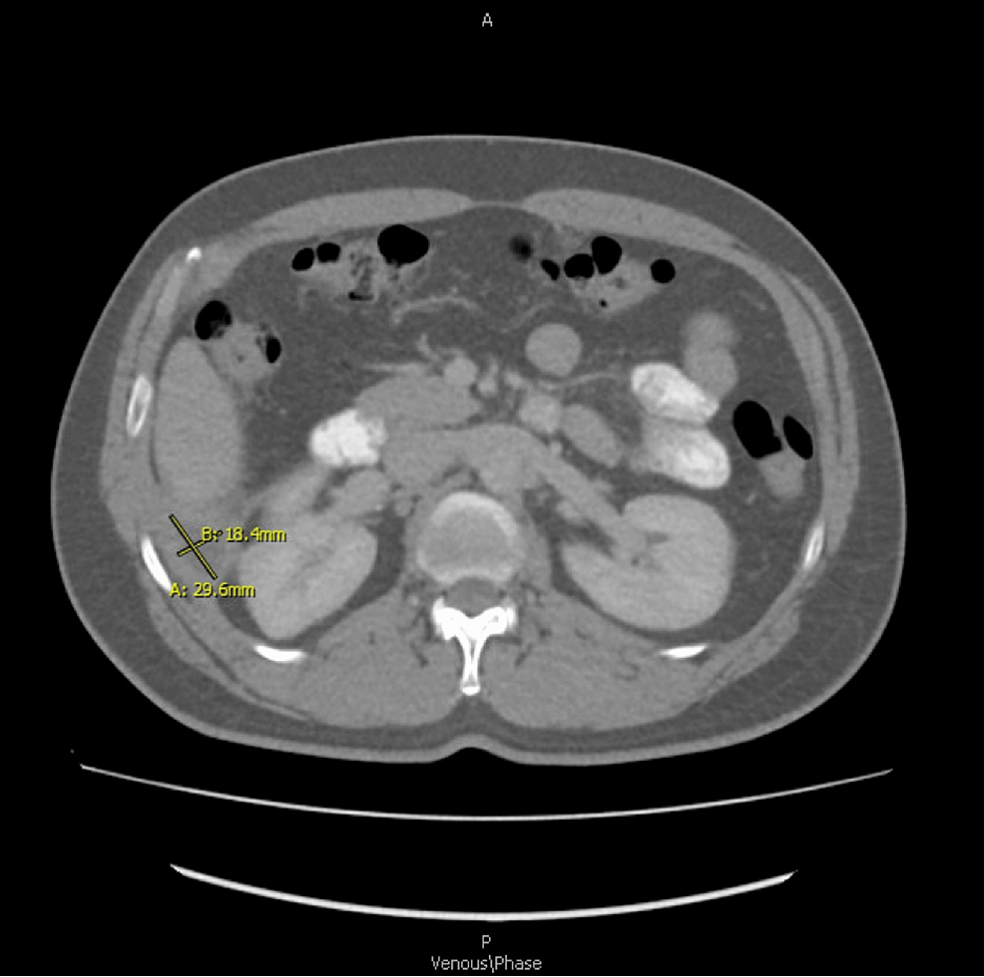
Equivocal CTAP imaging, axial (one year after surgery) representative of infra-hepatic abscess vs. potential retained spilled stones. Radiology at this time felt the findings were more consistent with abscesses. CTAP: computed tomography arterial portography
